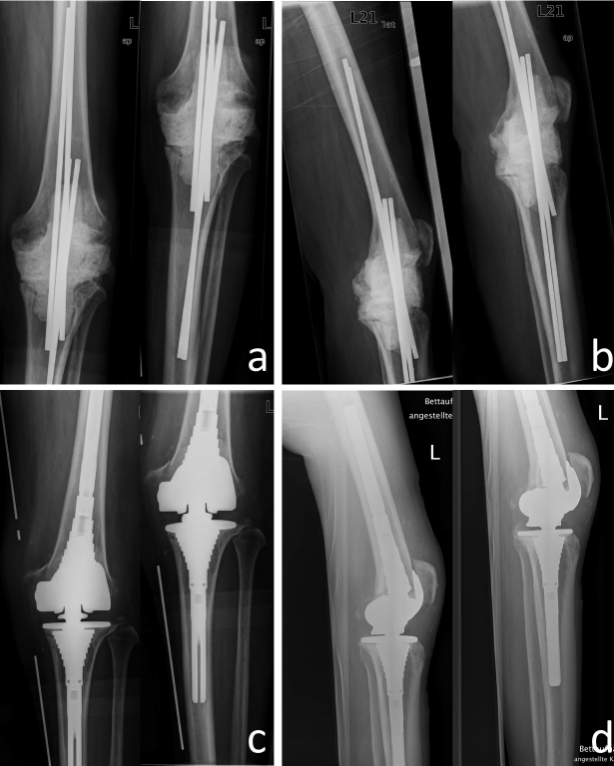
79-year-old patient with TKA infection left. a) Radiograph of the knee anterior-posterior and b) lateral after temporary arthrodesis with intramedullary fixation with titanium rods and cement mantle. c) X-ray knee anterior-posterior and d) lateral after re-implantation of a revision-TKR (Typ S-ROM, Company DePuy Synthes, West Chester, PA, U.S.A.)
